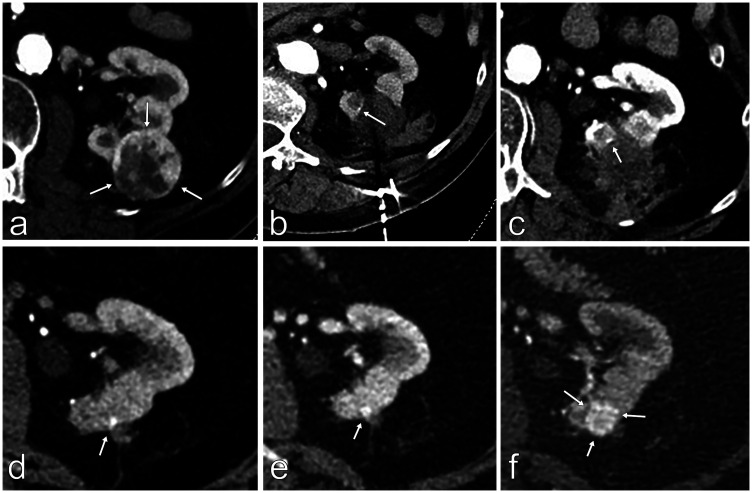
Example of a tumor treated with MWA. (a) Pretreatment CT showed a 3.5-cm tumor, m-RNS 9p points (arrows). (b) After treatment with 100 W (ablation time 10 min), a small part at the tumor border (arrow) was not fully treated. The antenna was repositioned, for further ablation (a further 3 min). (c) At the six-month follow-up, there was a high suspicion of residual tumor at the ablation margin (arrow). (d–g) The patient was followed at 1 year (d), 1.5 years (f), and 2 years (g) after ablation, verifying the successively growing residual tumor (arrow). This patient is currently planned for a second ablation session. CT, computed tomography; m-RNS, modified RENAL nephrometry score; MWA, microwave ablation.
